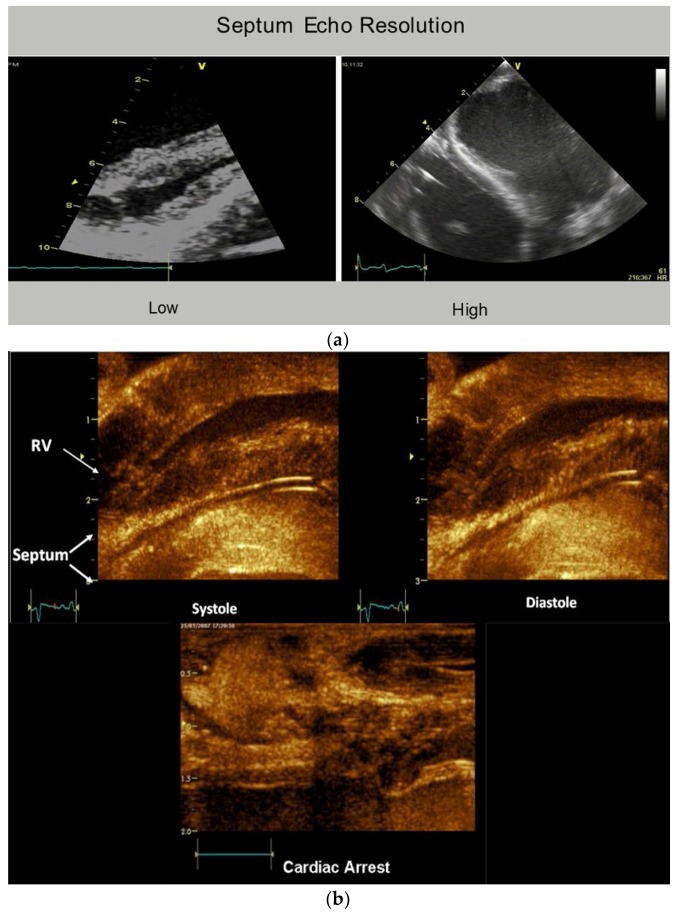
(a) Mid-septal hyperechogenic line shown in low and high-resolution echocardiogram; (b) septum high-resolution ultrasound image at transducer frequency (12 MHz), displaying its bilayer line with inner dimension structure of 100 to 150 μm. Working septum muscle fibers display different directionality on either side of septum line. This disappears, as does the echogenic line—when cardioplegia (cardiac arrest) stops contractile function. Reproduced form the reference [8] with Publisher’s permission.
